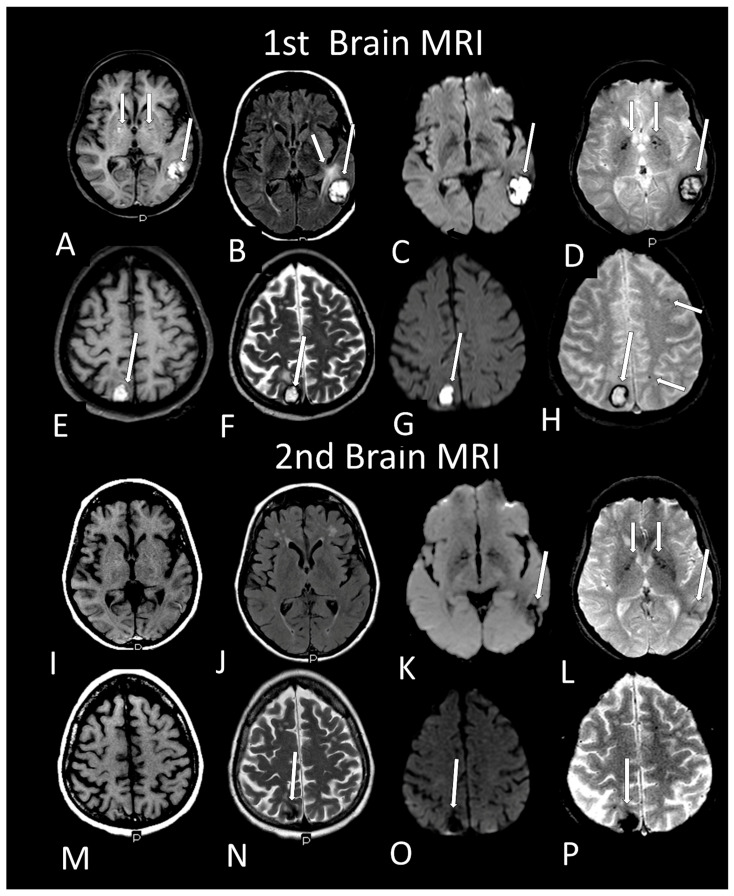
Case 27—On MRI, there is a subcortical hematoma located respectively in transition between left posterior temporal lobe and occipital lobe (long arrow A–D) with hyperintense methemoglobin inside on T1WI (A, long arrow), FLAIR (B, long arrow), DWI (C, long arrow) and T2* (D, long arrow) and with hemosiderin in the periphery being hypointense ring. There is vasogenic edema near the left temporal hematoma being hyperintense on FLAIR (B, short arrow). There is also microbleeding in pale globes (A,D, short arrows). Another cortical hematoma in the right parietal lobe (E–H, long arrow), with the same characteristics described above with methemoglobin inside and hemosiderin in the periphery. There are small dots of subcortical microbleeding in the left frontal and parietal lobes (H, short arrows). Two months later there was regression on MRI (I–P) of both hematomas in size, but deposition of hemosiderin remained being hypointense on all sequences [T1, (I and M); FLAIR, (J); DWI, (K,O, arrow); T2, (N, arrow); and T2* (L,P arrows)] and in the lentiform nuclei (L, small arrows).
